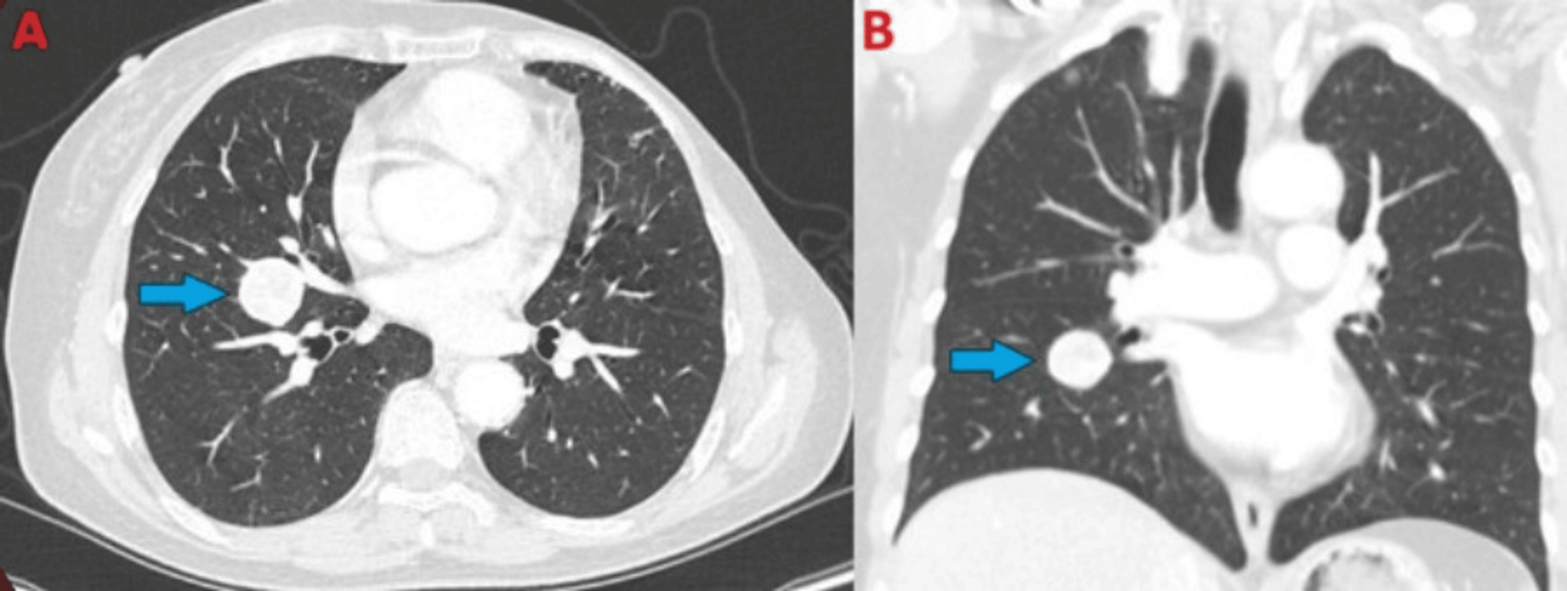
Chest CT (lung windows) demonstrating right circumferential lung nodule (blue arrows) measuring 2.4 x 2.5 cm in axial view (A) and coronal view (B).
